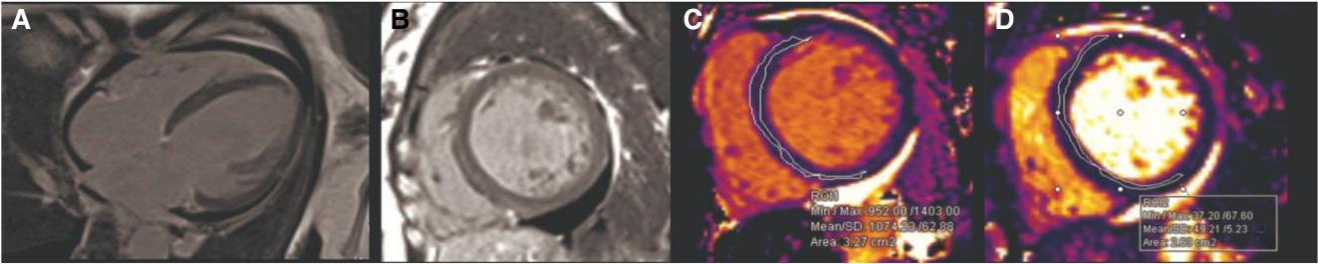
MRI of the heart showing (*A*) the longitudinal 4-chamber views demonstrate a dilated left ventricle with an end-diastolic volume of 126 mL/m². There is global hypokinesia observed on cine steady-state free precession images, with reduced left and right ventricular systolic functions of 20% and 22%, respectively. A pericardial effusion with a maximal thickness of 1.3 cm is also noted. (*B*) The late gadolinium short-axis image showed subtle intramyocardial enhancement, particularly in the septal and anterior walls. (*C*) and (*D*) Mid-ventricular septal measurements of T1 and T2 mapping demonstrate slight increases in T1 and T2 mapping values, respectively.
